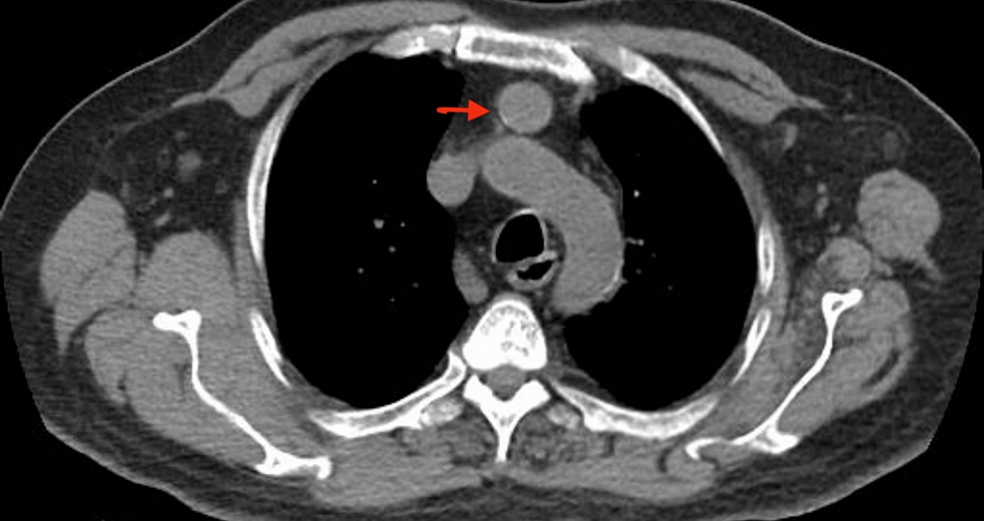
CT of the chest without contrast showing a well-circumscribed soft tissue nodule in the anterior mediastinum.
